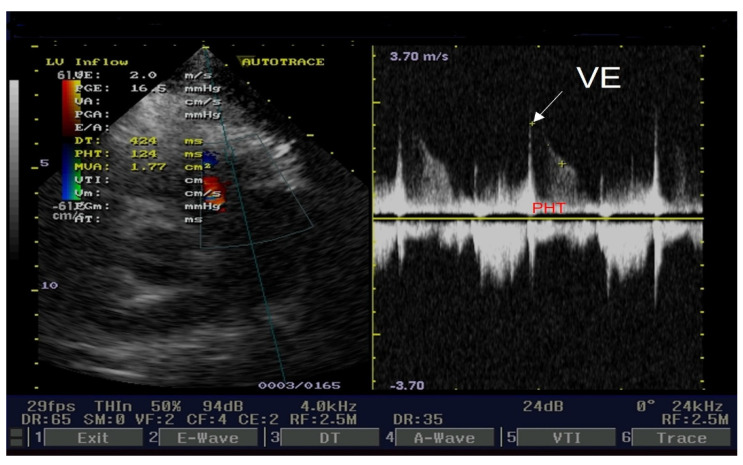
Echocardiography short axis view: PHT for pulmonary regurgitation.
